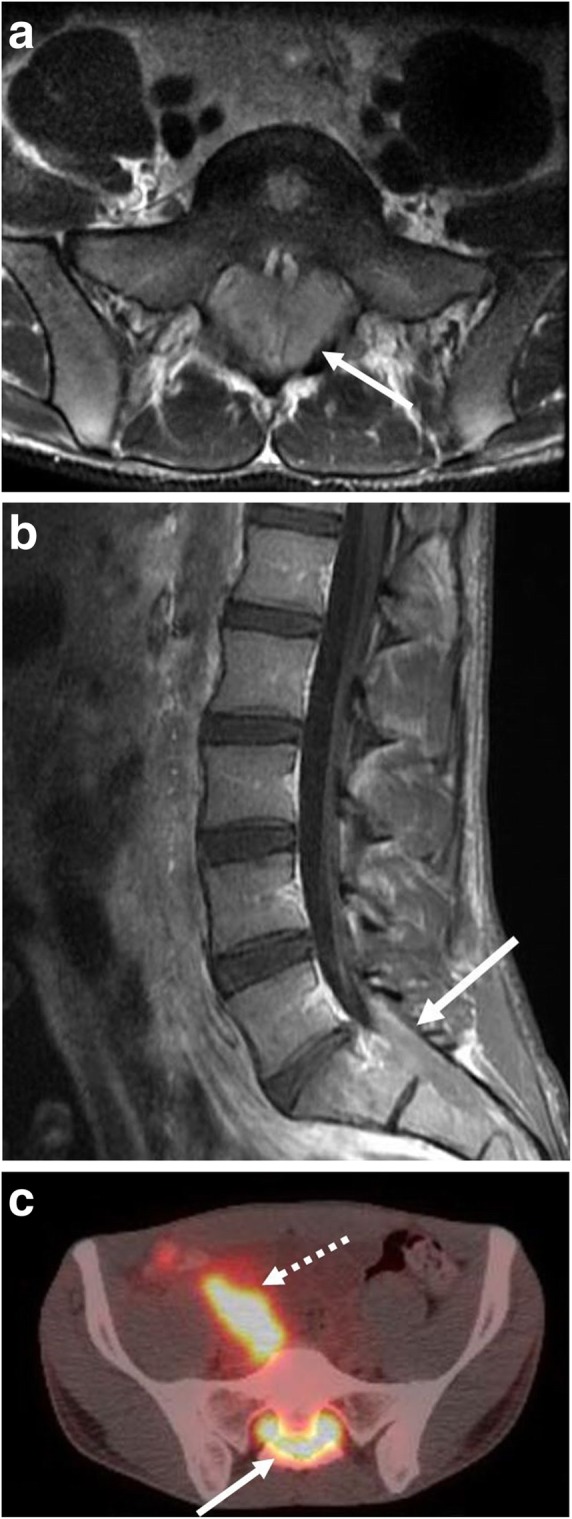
Central nervous system involvement. A 24-year-old male presenting with abdominal and lower back pain. a Axial T2-weighted and (b) sagittal post contrast images of the lumbar spine and sacrum demonstrate an enhancing soft tissue mass within the lower spinal canal (arrows) with extension into bilateral sacral neural foramina. c Fused axial PET-CT demonstrates associated hypermetabolic activity within the sacral mass (solid arrow). Involvement of the right retroperitoneum is also seen (dashed arrow)
